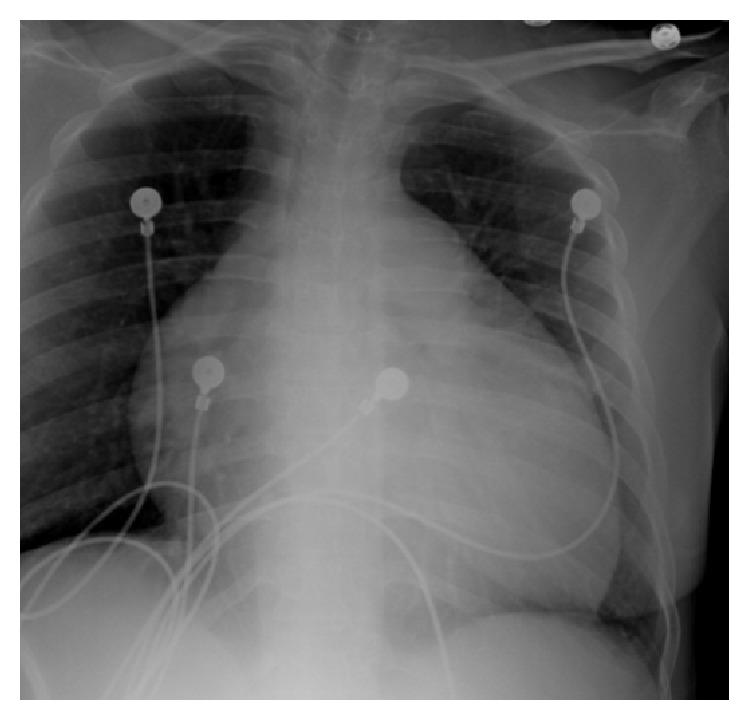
Chest X-ray image showing a significantly enlarged cardiac silhouette.
